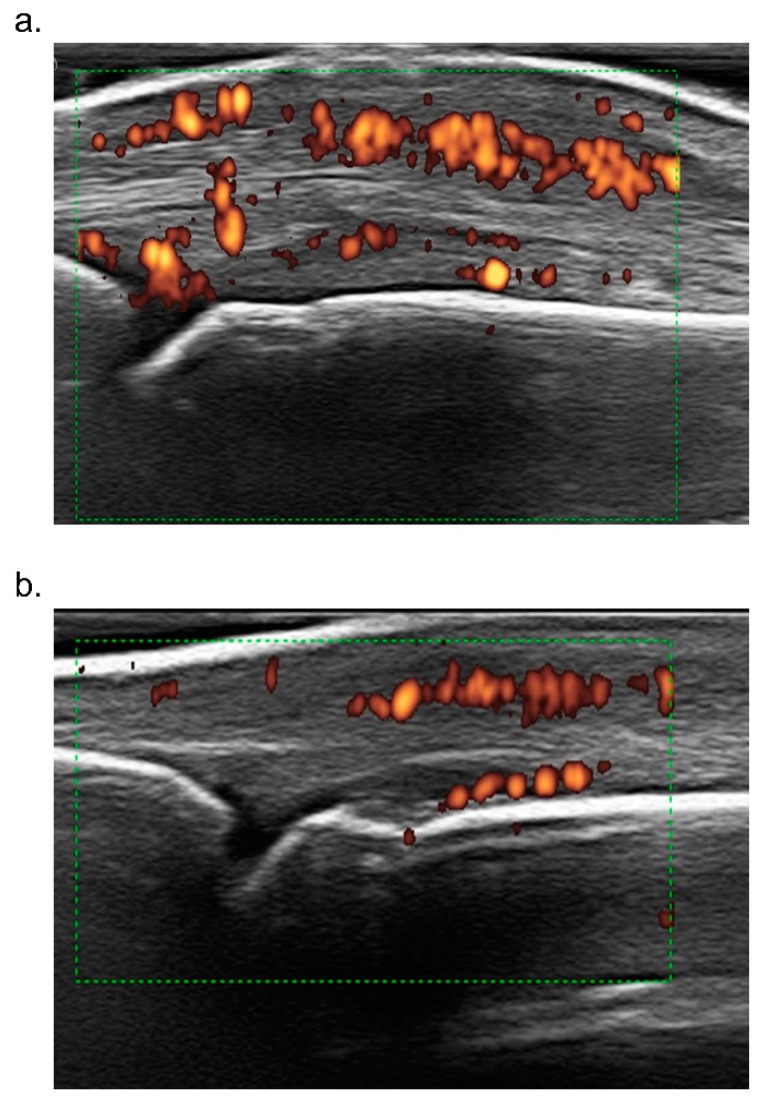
Ultrasound dorsal scan of the third metacarpophalangeal joint of the right hand of our patient. Swelling of the periarticular tissues, power doppler signal (red dots) of the joint and in the peritendon of the extensor of the finger were more evident at baseline (a). After 6 months, the power doppler signal and the periarticular swelling were considerably reduced (b).
